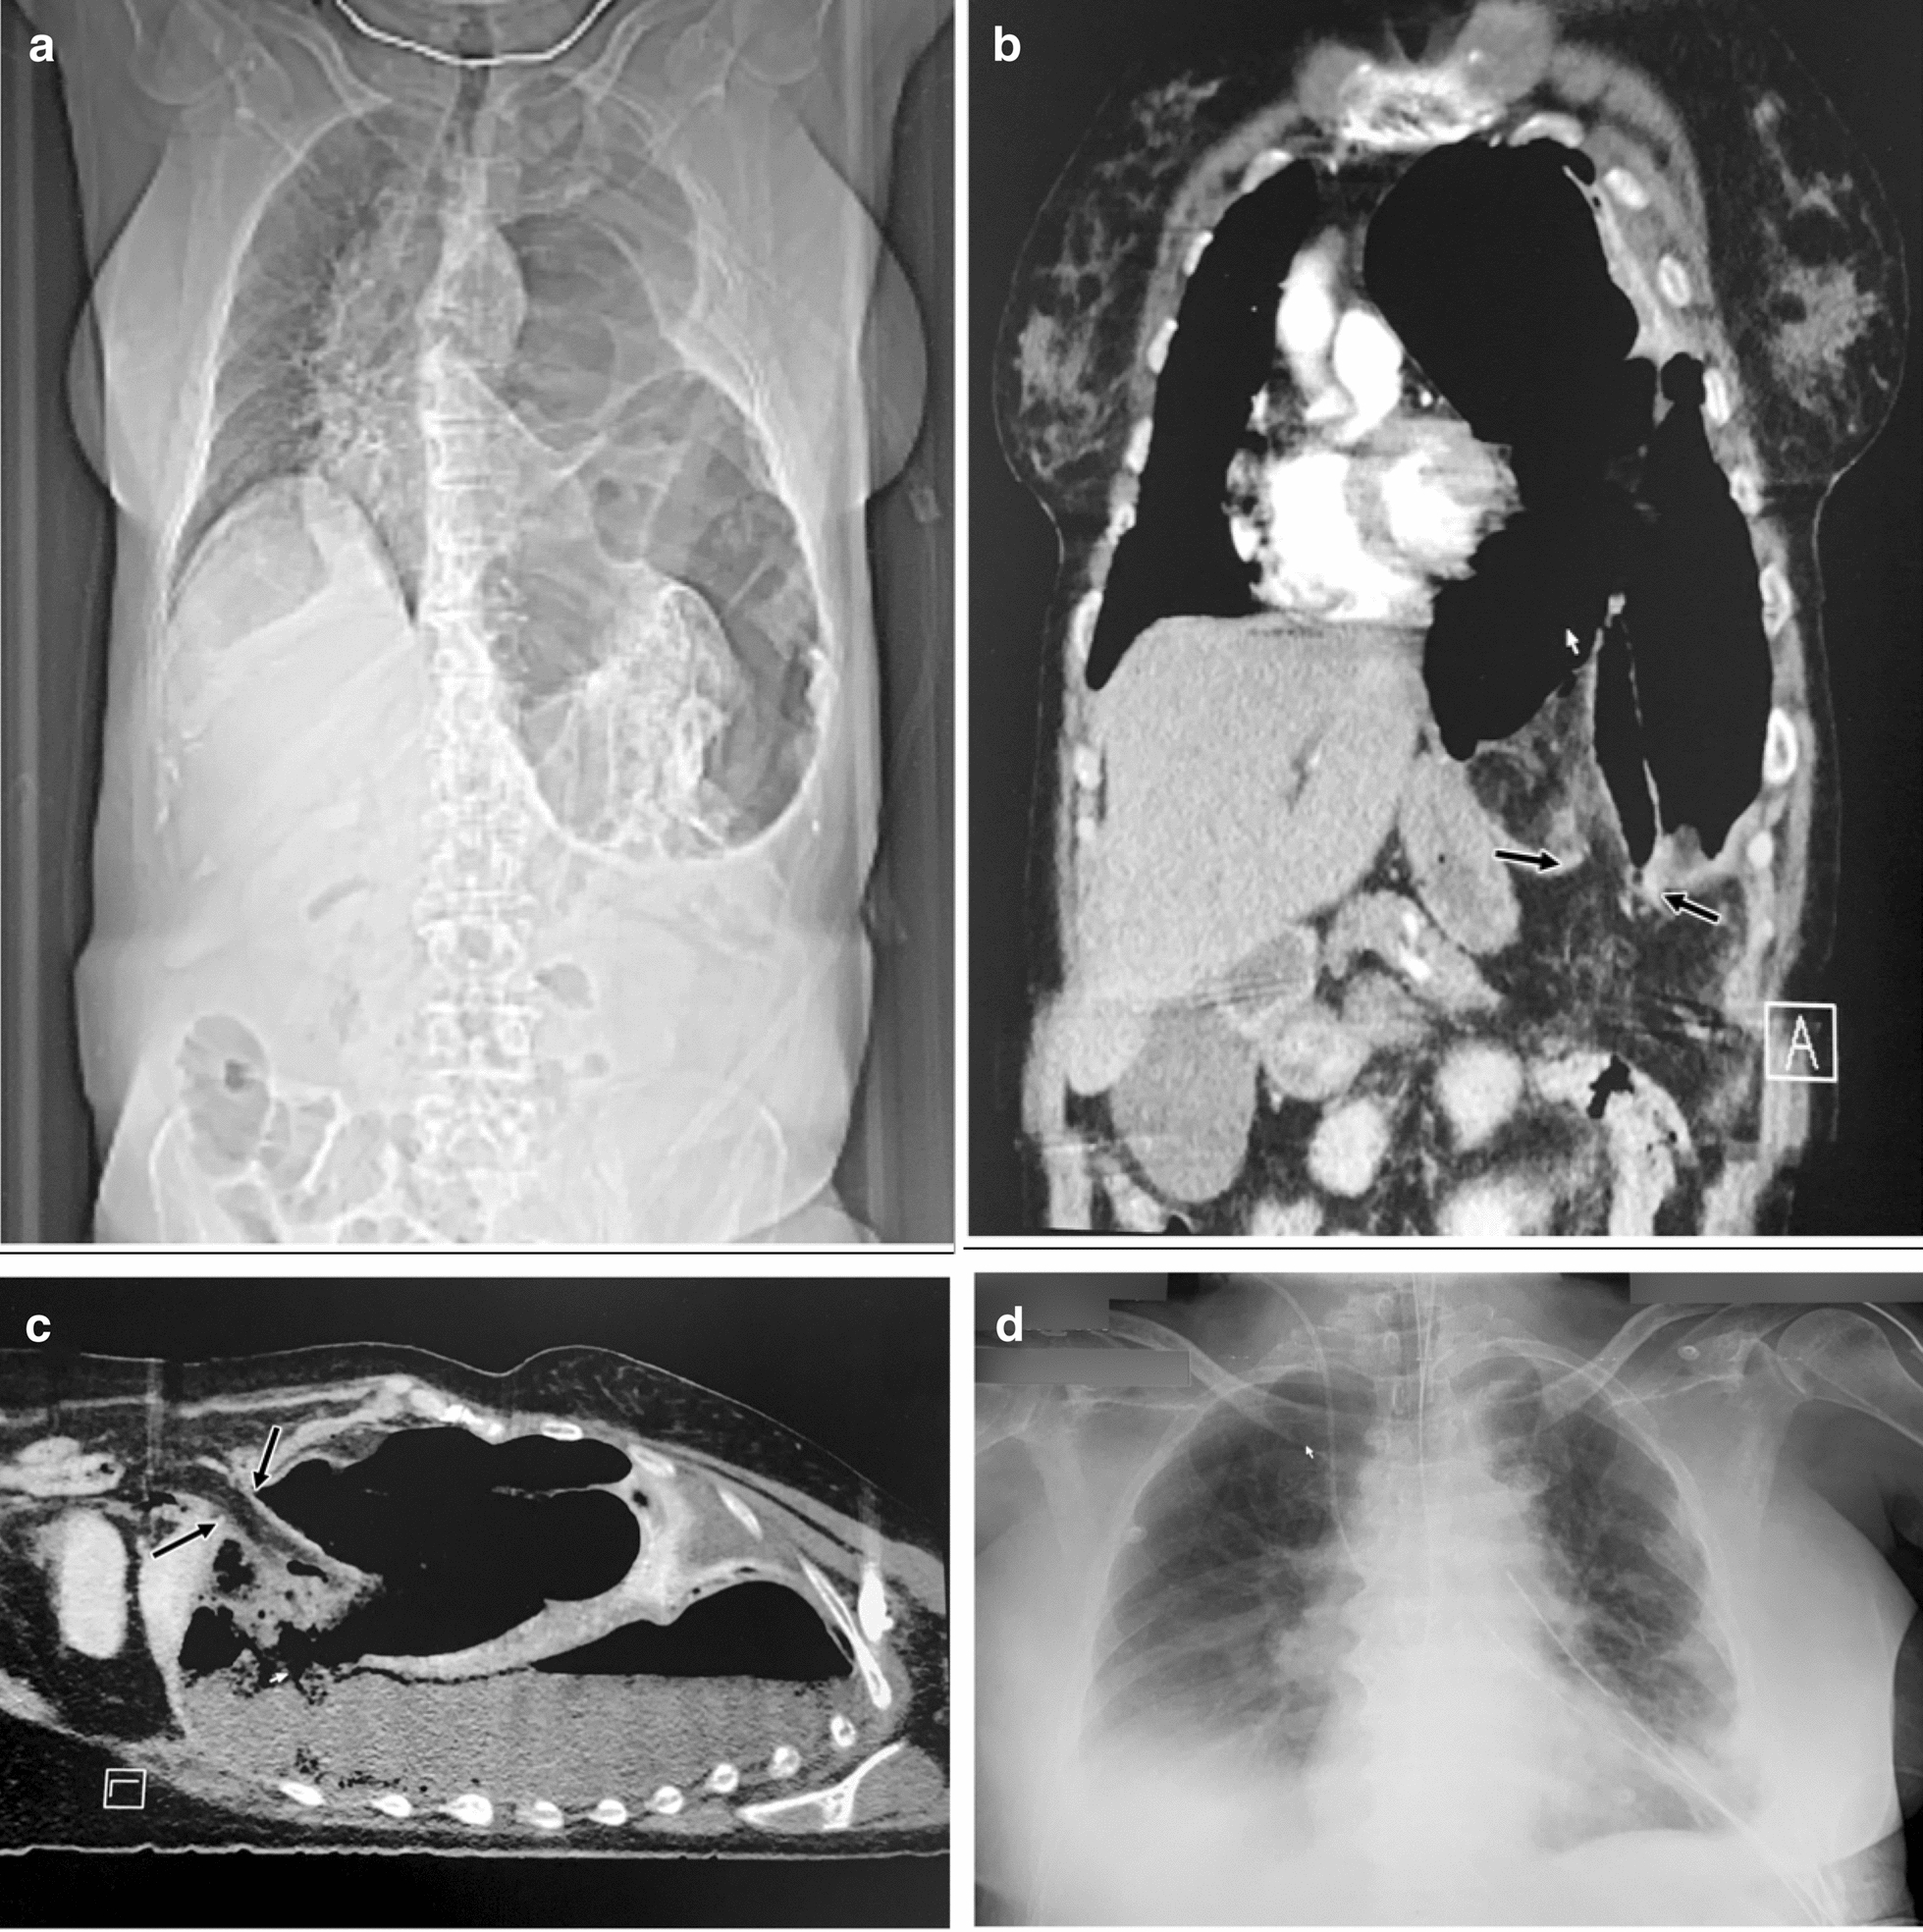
Radiological imaging on day of admission. Thoraco-abdominal CT scan with localizer of carried out CT scan (a) and representative CT scan images (b, c): enterothorax with splenic flexure prolapse through an old undetected traumatic rupture of the left diaphragmatic center, consecutive colic incarceration (b + c; arrows) with ischemia, rupture of the colon into the left pleural cavity with feco-pneumothorax under tension, cardiac depression due to mediastinal shift to the right and sepsis secondary to left pleural empyema (c), inversed diaphragm and midline shift to the right (a + b). Post-surgical chest X-ray examination (d): re-expanded left lung and reversal of the mediastinal shift with reconstructed diaphragm
